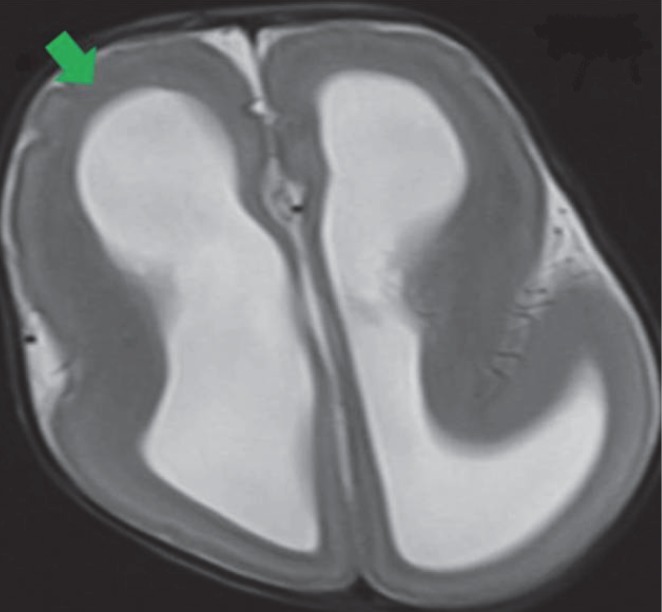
MRI axial T2 weighted images show reduction in normal sulcation smooth agyric cortex (green arrow) with hydrocephalus in type I Lissencephaly
